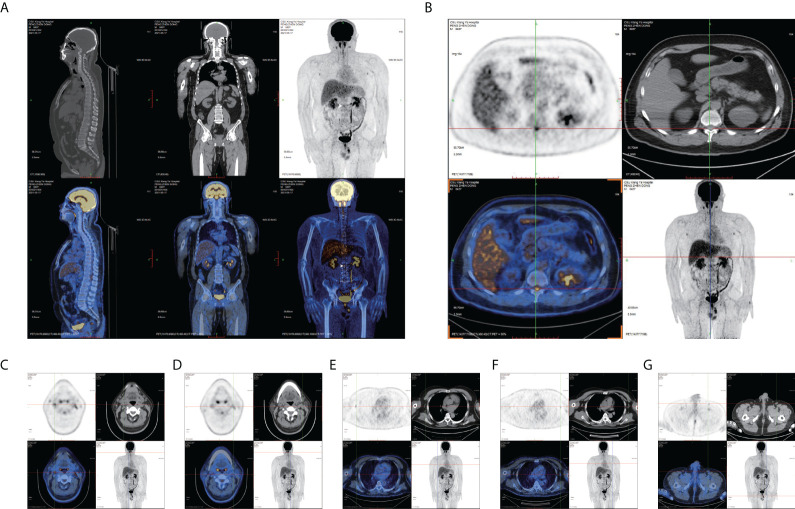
FDG PET images. (A) Sagittal and coronal PET/CT show a high concentration in the thoracolumbar segment (white arrow). (B) Axial PET/CT shows an extremely abnormal hypermetabolism of FDG near the T12 thoracolumbar segment (maximum standardized uptake value: 6.321). Axial PET/CT in (C, D) bilateral cervical lymph nodes, (E, F) bilateral axillary lymph nodes, and (G) epididymis.
